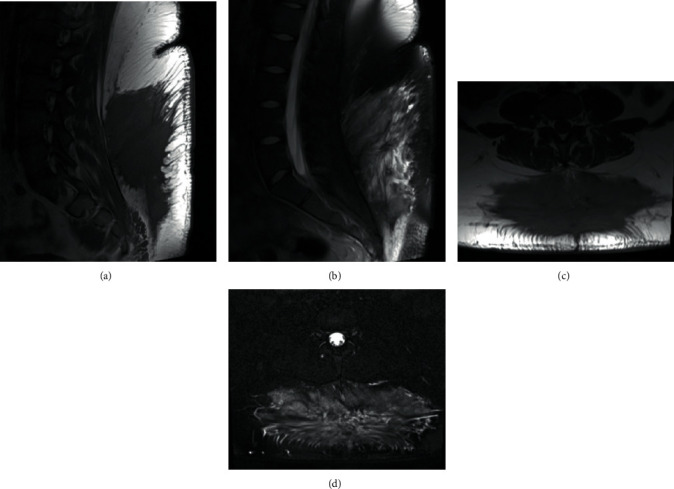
Representative 9-month postoperative 1.5 Tesla MRI images. (a) T1 sagittal (TR 500 ms, TE 17 ms). (b) T2 FS sagittal (TR 4,770 ms, TE 83 ms). (c) T1 axial (TR 600 ms, TE 19 ms). (d) T2 FS axial (TR 6,580 ms, TE 100 ms). These images revealed a large T1 hypointense, T2 heterogeneously hyperintense, and enhancing lesion with irregular borders, measuring approximately 21.5 × 7 × 15 cm (transverse × anteroposterior × craniocaudal). There was a central stellate/spiculated portion of the lesion, which was a relatively nonenhancing region and suggestive of an area of postsurgical scarring. Surrounding this region was a more T2 hyperintense multilobulated appearing component. No other lesions were seen. FS = fat suppression, TR = repetition time, and TE = echo time.
